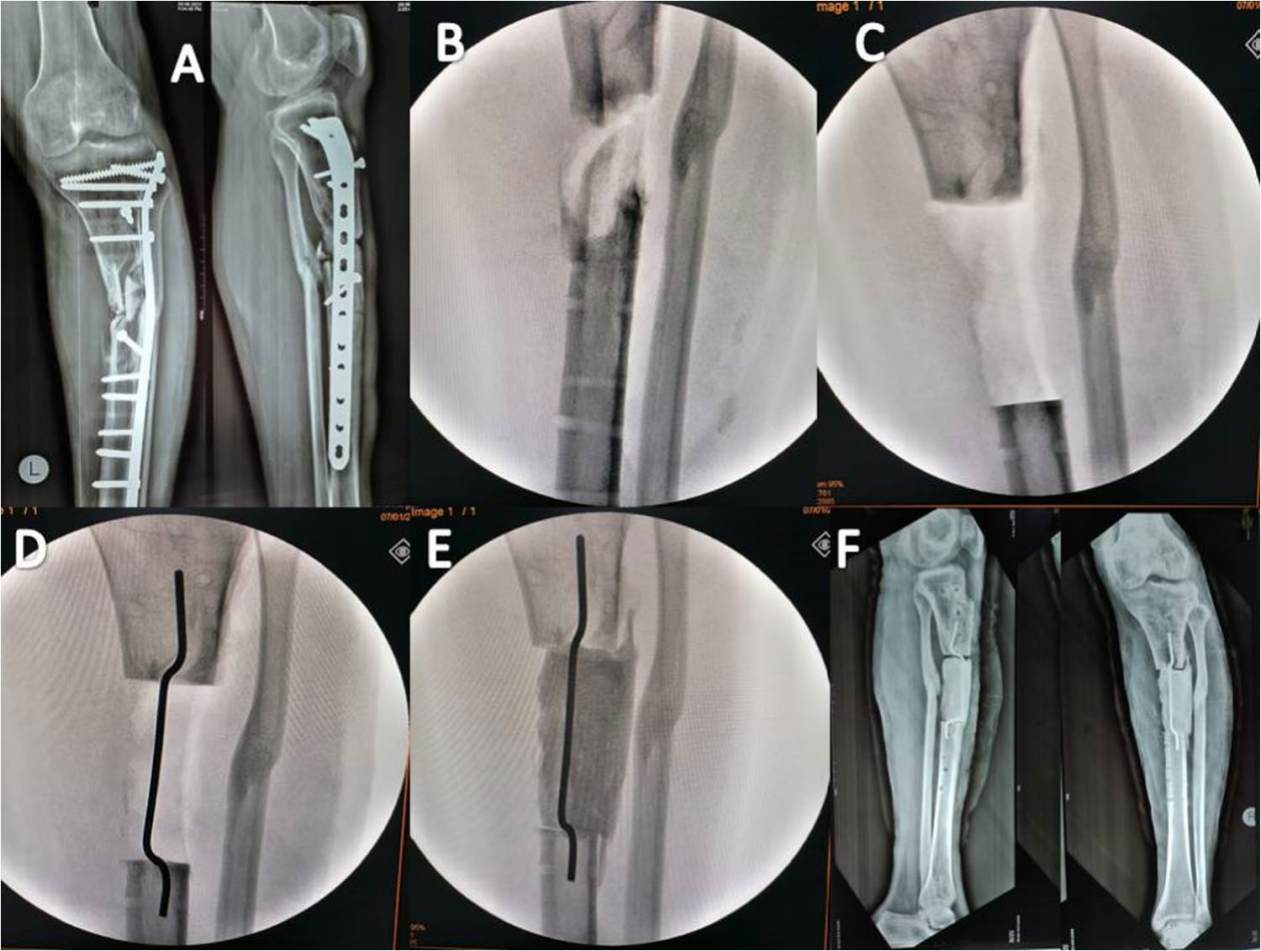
Phase 1 of surgical debridement and stage 2 of management of the dead space. A Ap and lateral X-ray of infected non united proximal tibia fixed with plate with residual deformity. B After removal of the hardware. C Removal of the necrotic bone. D, E Obliteration of the dead space with cement spacer applied on single double leveled K-wire. F Postoperative Ap and lateral X-ray
